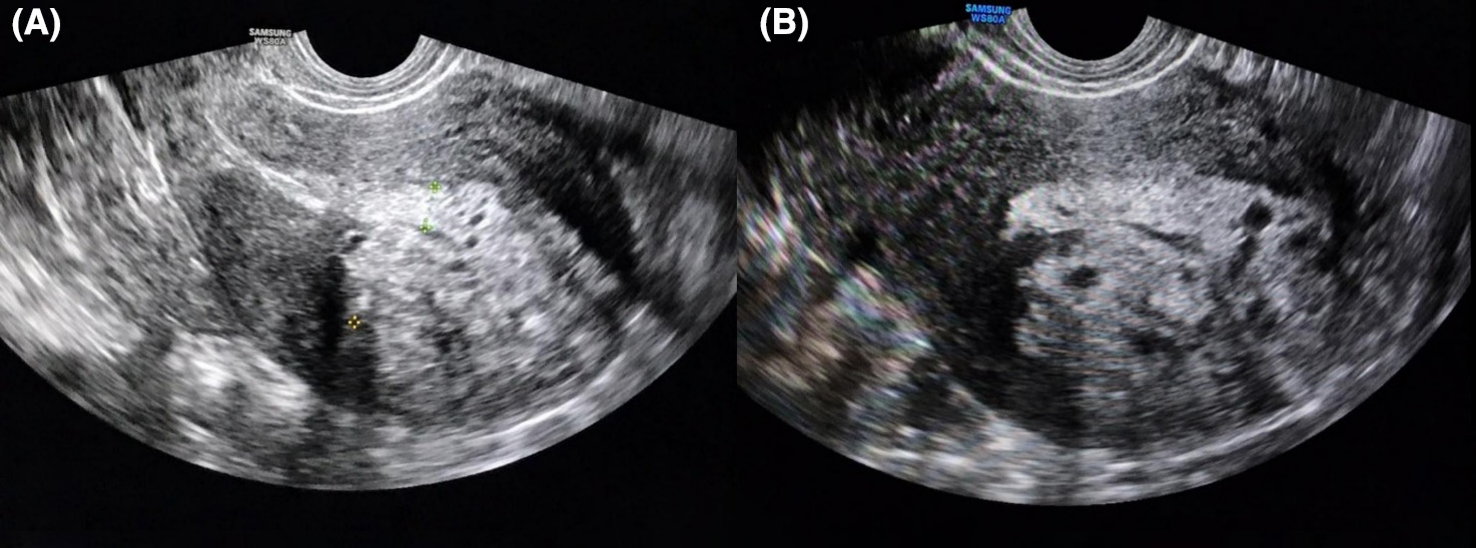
Transrectal sonography reveals complex uterine lesion. (A) Transrectal sonography reveals a globular uterus with asymmetrical myometrial thickening and irregular junctional zones. A lobulated heterogeneous hyperechoic mass‐like lesion, measuring approximately 75 × 57 × 55 mm, is observed at the anterior of the uterus. The lesion displays a few cystic changes and appears to be located at the junctional zone, invading the fundal deep myometrial region. (B) A close‐up view of the hyperechoic mass‐like lesion, showing its lobulated appearance and areas of cystic changes, further highlighting the complexity of the condition.
